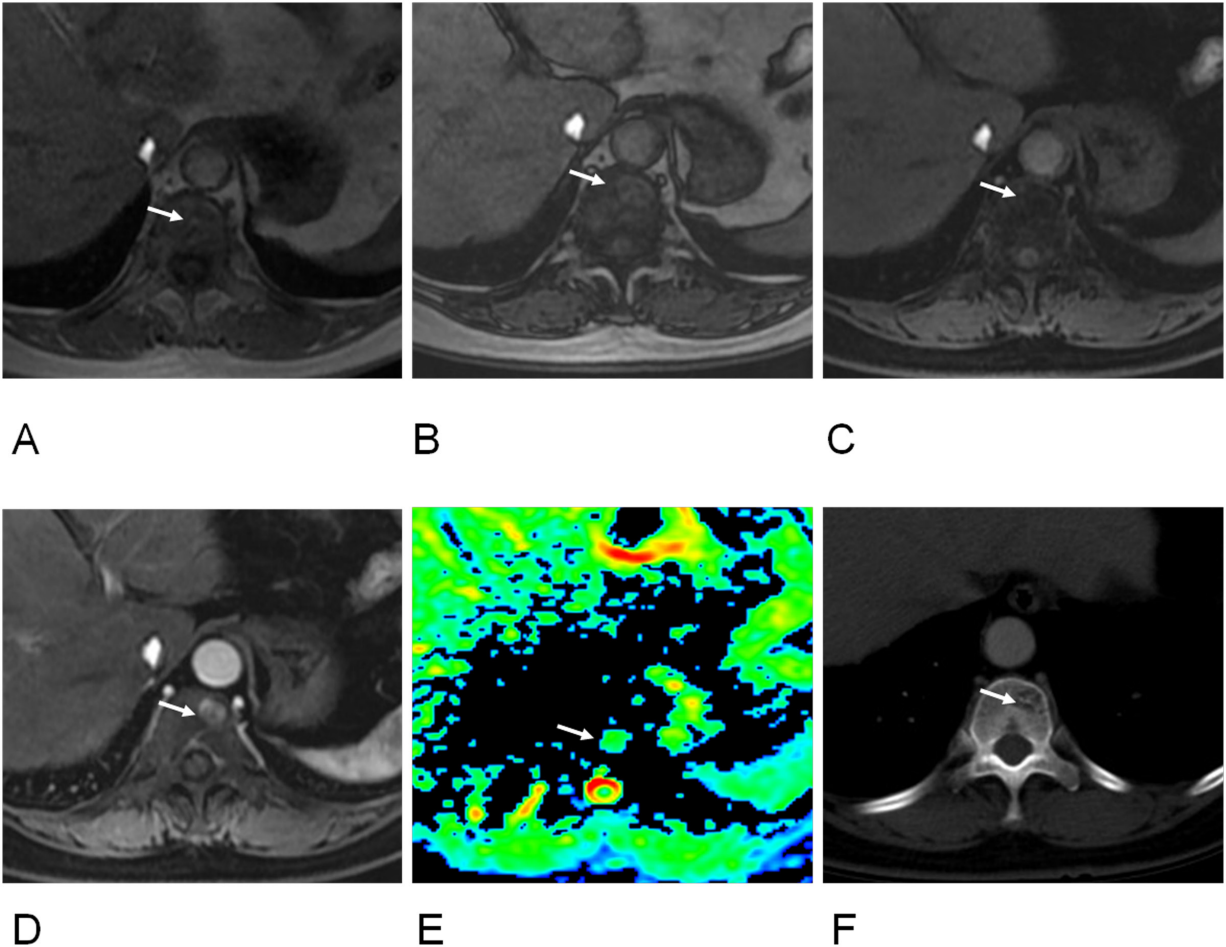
60-year old women with atypical hemangioma of T11 vertebra (A) T1-weighted image showed an isointense signal lesion on T11 vertebra (arrow). (B) Out-phase image showed a hyperintense signal lesion (arrow), ratio of SI loss between in- and out-phase was 0.35. (C) Fat suppressed (FS) T1-weighted images showed an isointense signal (arrow), ratio of SI loss between T1 and FS T1 was 0.74. (D) Early enhanced image showed hyperintense signal (arrow) (enhancement ratio, 4.47; SIR, 2.21). (E) Apparent diffuse coefficient (ADC) was 1.13 x10^-3^ mm^2^/s (arrow). (F) CT image revealed a hypo-dense lesion with internal striated appearance matching with hemangioma (arrow).
